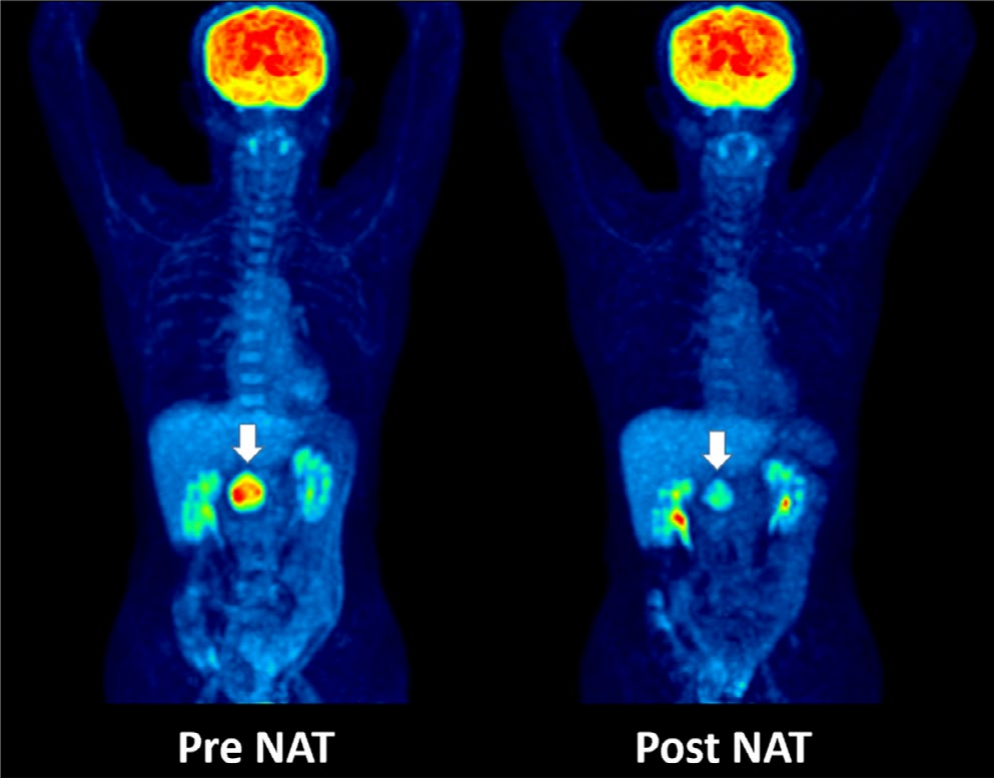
Representative FDG PET/CT examination before and after neoadjuvant therapy (NAT) revealed a significant decrease in FDG uptake in the tumor (arrow)
